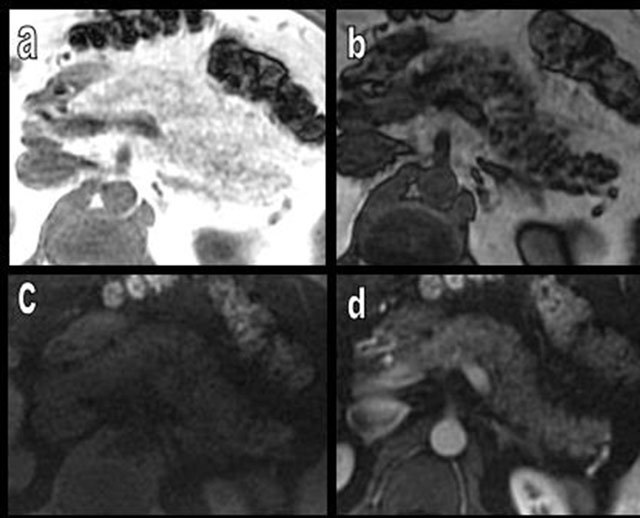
MRI of the pancreas performed to exclude choledocholithiasis in a patient who has suffered from acute pancreatitis two months before. Transverse in- and out-phase MR images respectively show a typical global hyper- (a) and hypointensity (b) of the pancreas. A T1-weighted image with fat suppression (c) confirms hypointensity of the pancreas due to massive FI. A T1-weighted image with fat suppression after gadolinium enhancing (d) shows homogenous enhancement of the entire pancreas.
